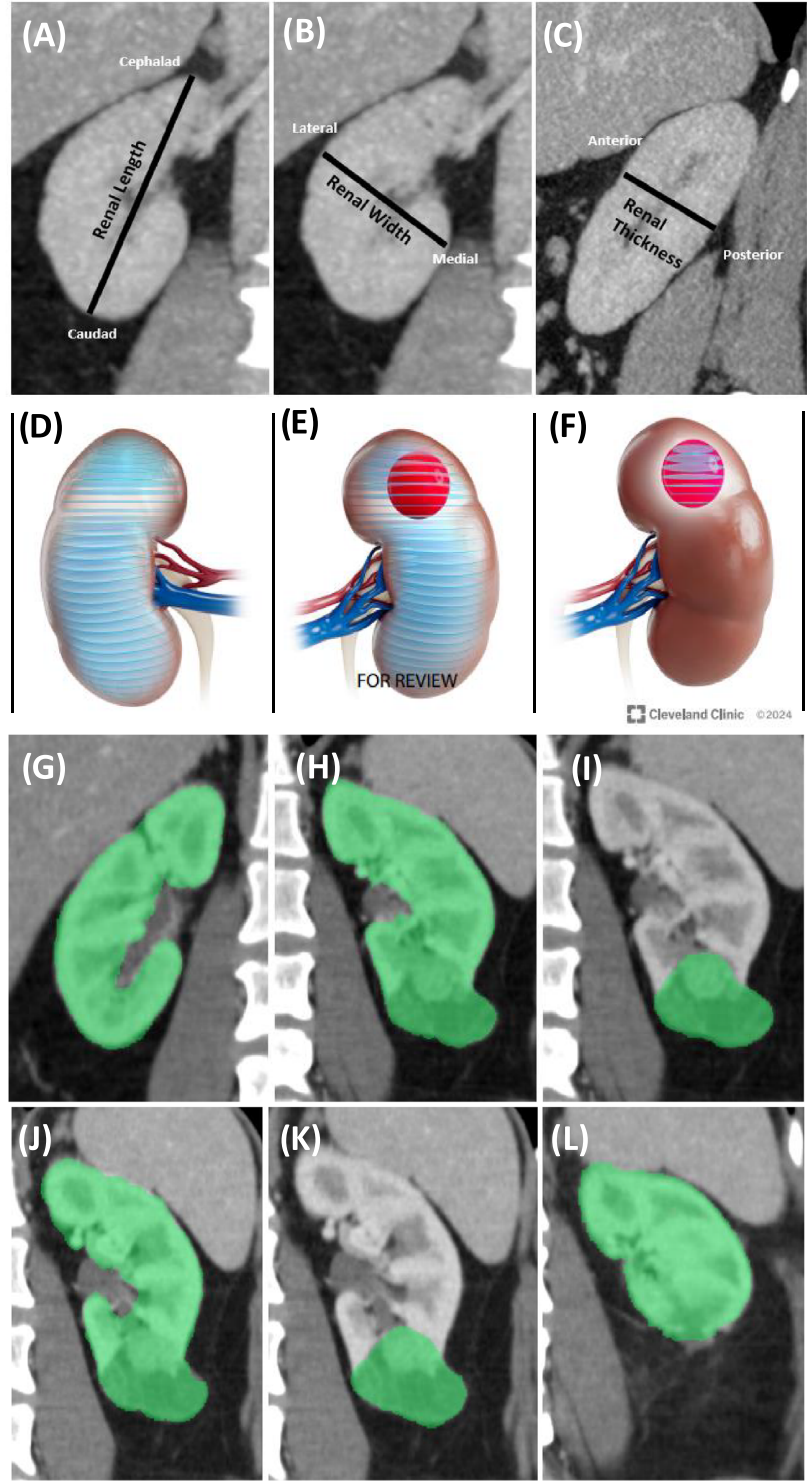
Various approaches to perform parenchymal volume analysis to obtain renal functional metrics, such as split renal function (SRF) and percent parenchymal volume preserved (PPVP). (A–C) Estimation of renal parenchymal volume based on linear measurements of the length, width and height (LWH) as described by Schober et al.^16^ and Feder et al.^17^ (A) Linear LWH measurements showing length (cephalad to caudad, coronal view); (B) width (lateral to medial, coronal view); (C) thickness (anterior to posterior measurement, sagittal). (D–F) Free‐hand scripting segmentation procedure utilized in CT/MRI volumetric analyses to estimate differential parenchymal volume and split renal function. Transverse measurements of areas are made at 3 mm intervals and then summed to give a direct estimate of the parenchymal volumes in the ipsilateral versus the contralateral kidney, as described in Mir et al.^18^ (D) segmentation to obtain the parenchymal volume of the contralateral kidney. (E) Segmentation to obtain the total volume of the ipsilateral renal parenchyma and tumour. (F) Segmentation to obtain the volume of the tumour alone, which is then subtracted from (E) to determine the preoperative ipsilateral parenchymal volume. (G–I) Semi‐automated software‐derived parenchymal volume analysis (PVA) to estimate the SRF (Fujifilm medical systems) as described in Rathi et al.^6^ (G) Measurement of the volume of the contralateral kidney. (H) Volume of the ipsilateral kidney + tumour. (I) Volume of the tumour alone, which is then subtracted from (H to determine the ipsilateral parenchymal volume. SRF is based on the relative amounts of parenchyma on each side normalized by total parenchymal volume. (J–L) Use of semi‐automated software to determine PPVP following PN as described in Kazama et al.^19^ (J) Tumour plus parenchyma. (K) Tumour alone. (L) Parenchyma present in postoperative state. These techniques demonstrate the evolution of PVA calculation methods as each iteration improved the technique of determining SRF and PPVP. With all these methods it is important to exclude cysts, central sinus, collecting system and other unrelated structures when measuring the parenchymal volumes on each side before and after surgery.
